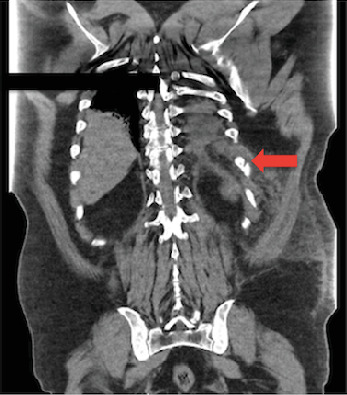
Computed tomography (CT) of the chest coronal view on admission shows minimally displaced transverse fracture of the 10^th^ rib (red arrow).
